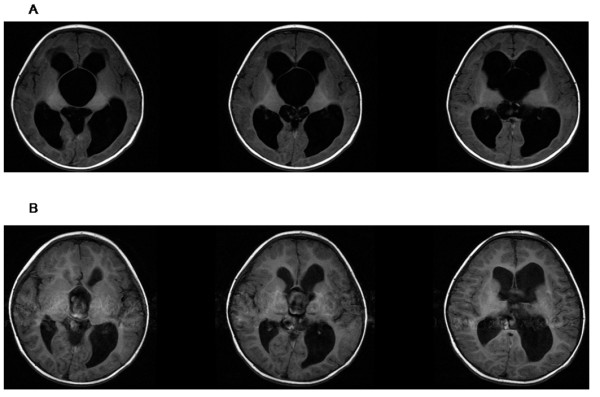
Axial magnetic resonance images obtained in a patient who harbored SSC and hydrocephalus. A: Preoperative axial T1-weighted image (male, 1 year old). B: Postoperative axial T1-weighted image (25 months later).
